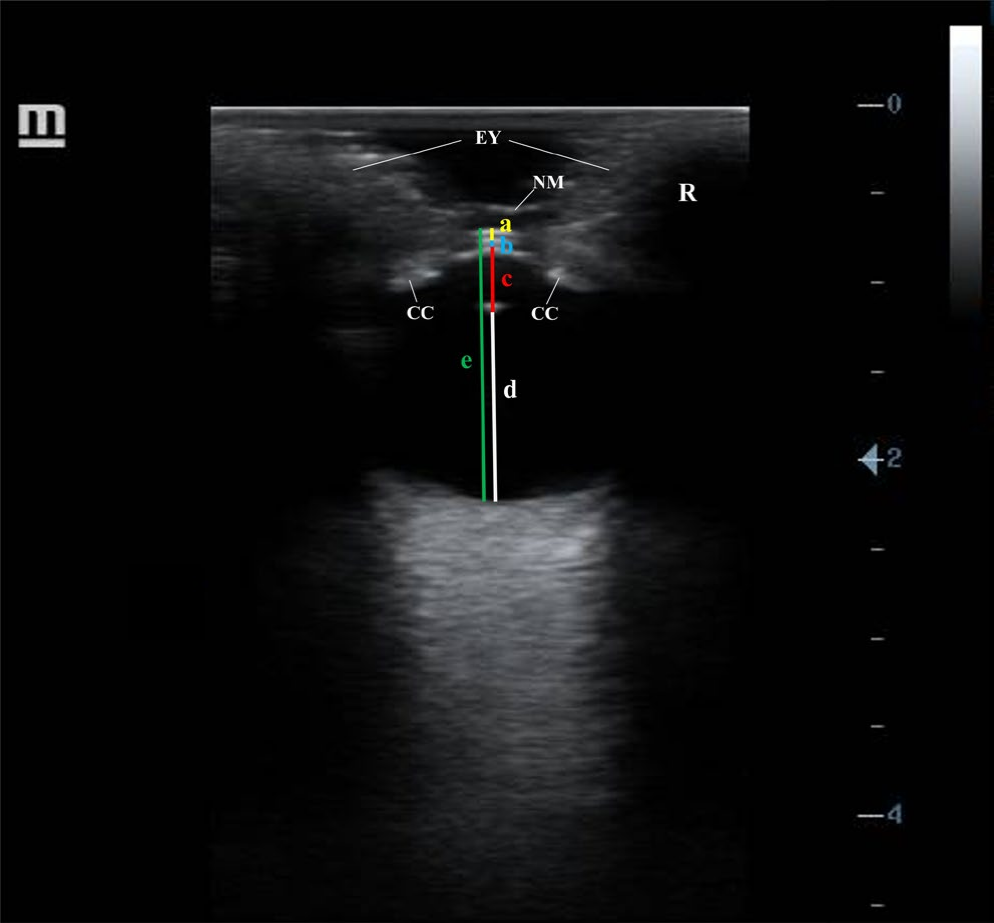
Ultrasonographic ocular biometry assessment in a Greater Caribbean manatee (
